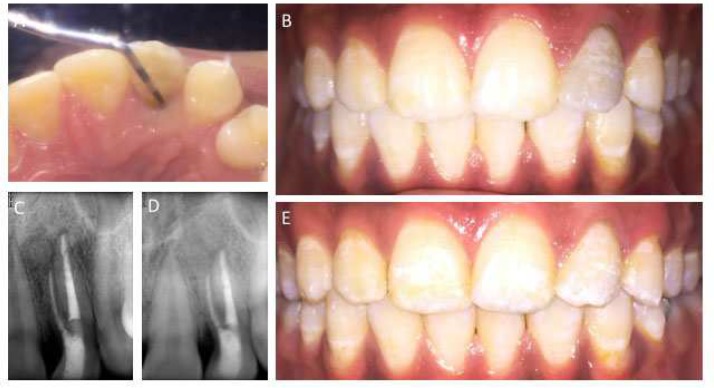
A)* 2 year follow-up, reduced probing depth; *B)* Discoloration of the crown was present; *C)* 1 year follow-up radiograph; *D)* 2 year follow-up radiograph; *E)* Walking bleach was used to remove discoloration
